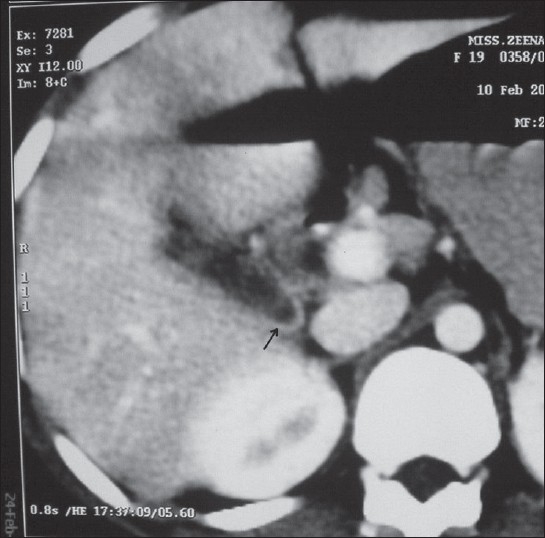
CT scan of hepatobiliary system
